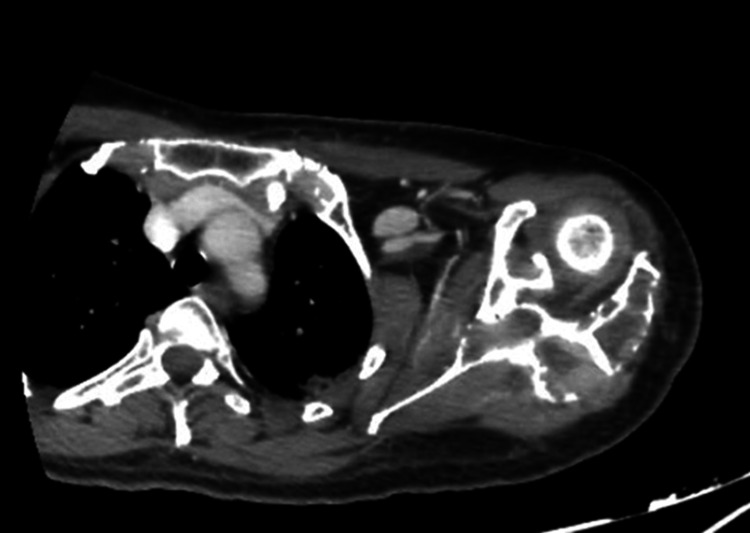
Axial follow-up CT of the shoulder showing marked reduction in the size and density of the previously observed osteolytic lesion, with partial re-ossification.
